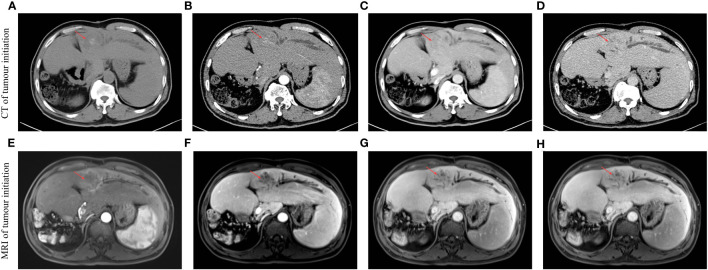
Multistage liver-enhanced CT and gadoxetate disodium-enhanced MRI scans of the liver showed that S3 and S4 occupied the liver at the first visit. The red arrow indicates an intrahepatic tumor. (A) Initiation-CT image in the period of non-contrast scan. (B) Initiation-CT image in the period of arterial phase. (C) Initiation-CT image in the period of portal vein phase. (D) Initiation-CT image in the period of delay phase. (E) Initiation-MRI image in the period of arterial phase. (F) Initiation-MRI image in the period of portal vein phase. (G) Initiation-MRI image in the period of non-contrast scan. (H) Initiation-MRI image in the period of hepatobiliary phase.
